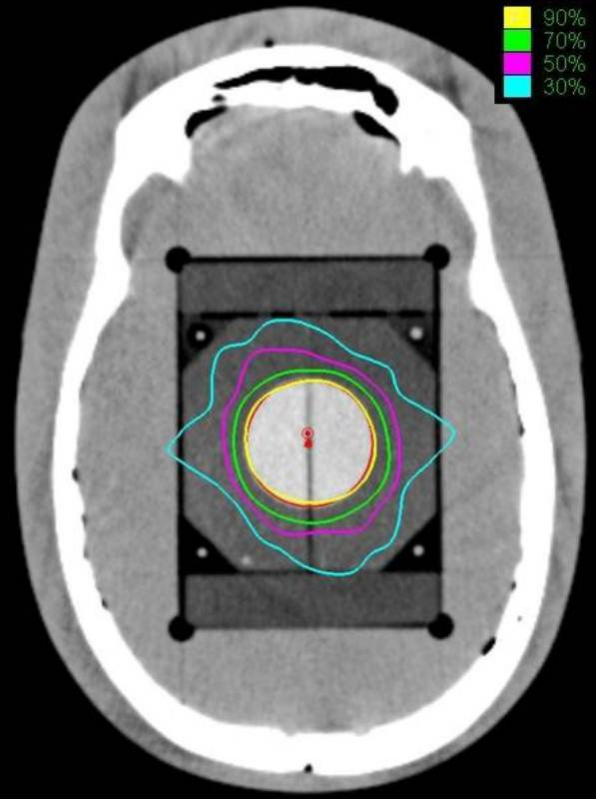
An example dose distribution by the optimal collimator (35 mm collimator) in the axial CT image for PTV1.
